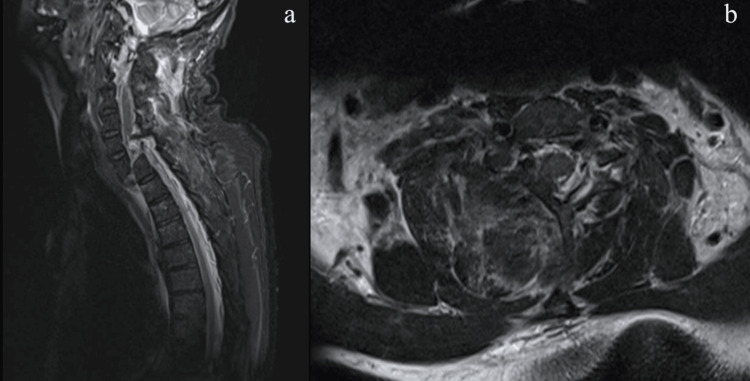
Preoperative sagittal and axial T2-weighted MRI. Preoperative sagittal and axial T2-weighted magnetic resonance images with sagittal (a) and axial (b) cuts showing significant cord compression and complete lithesis of C7-T1 with C7 disc herniation.
